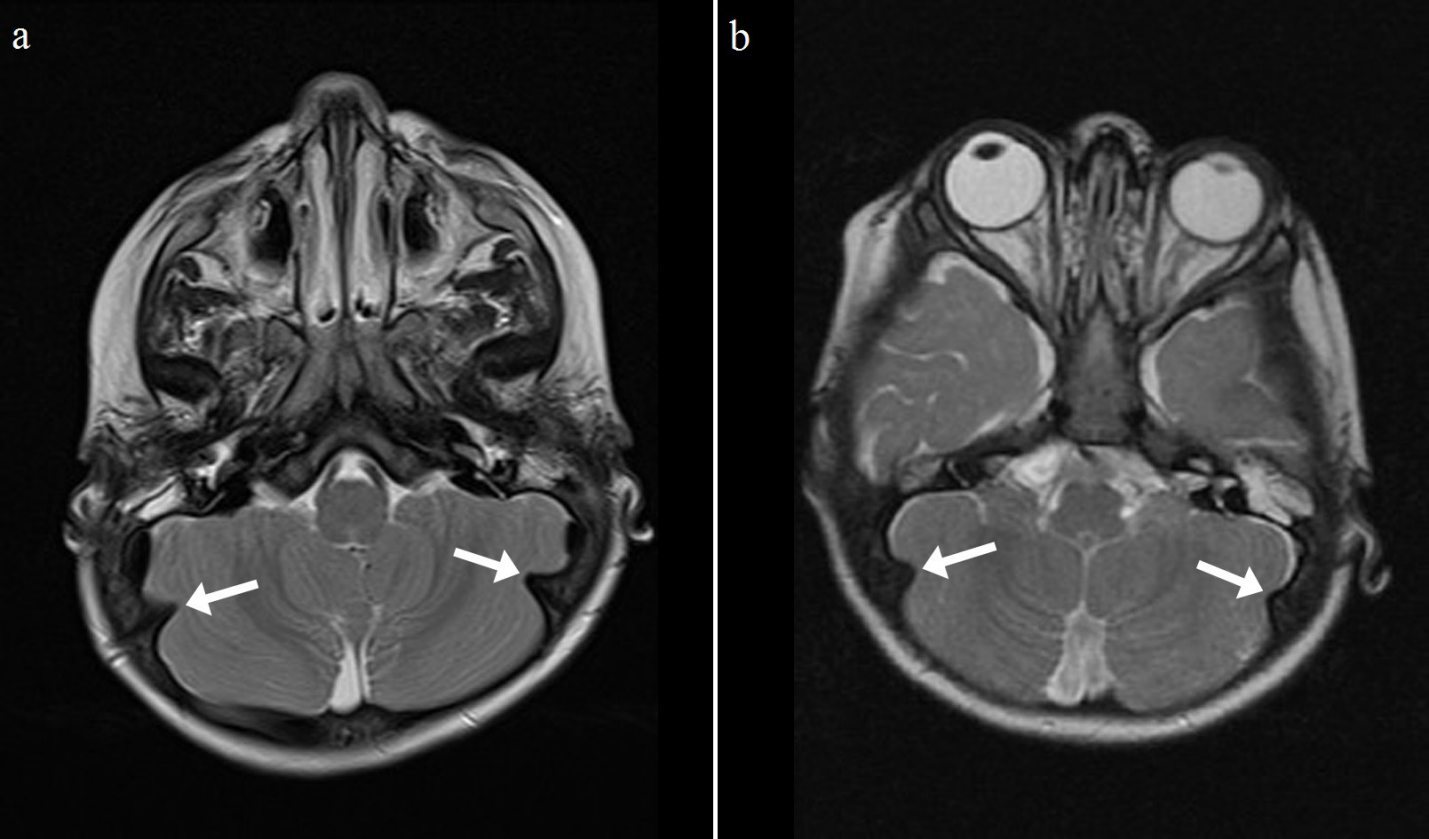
Axial T2 (a-b) weighted MR images show bilateral IHOMs (white arrows) of 2 different patients with MPS type VI. (a: 26 months old, b: 18 months old).
